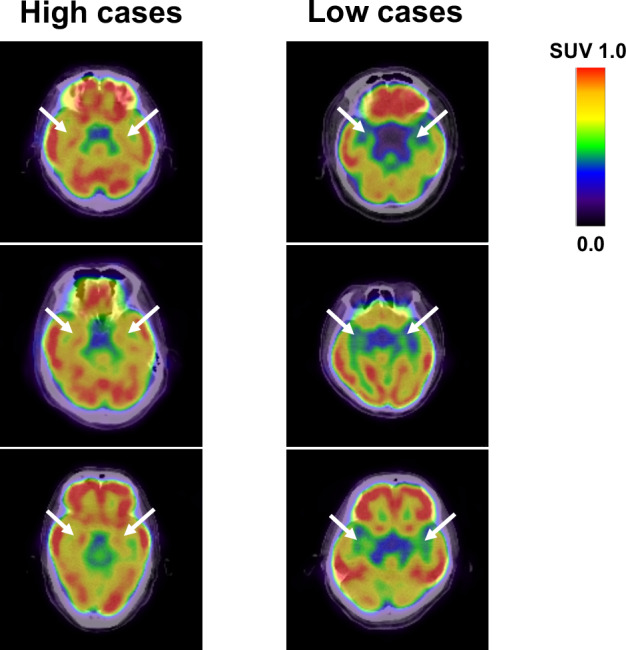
Representative FDG-PET/CT images of 6 cases. Axial views of the brain FDG/PET imaging in 3 cases with high amygdalar metabolic activity (left panels) and 3 with low activity (right panels). SUV standardized uptake value.
